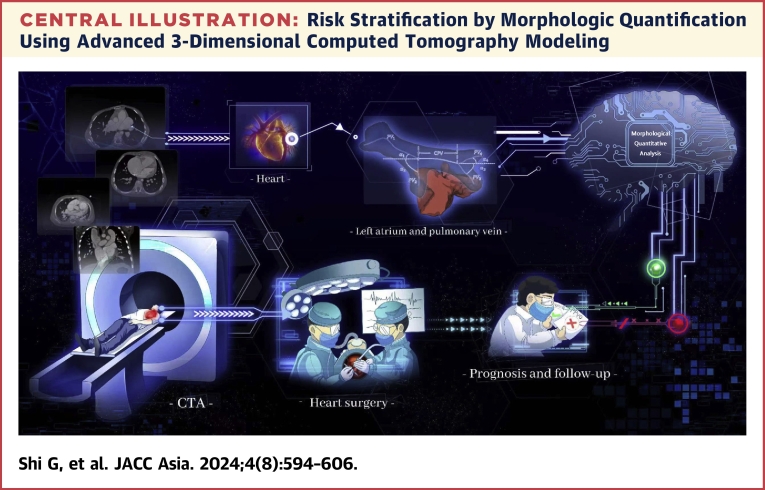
Risk Stratification by Morphologic Quantification Using Advanced 3-Dimensional Computed Tomography Modeling Morphologic quantification derived from advanced 3-dimensional computed tomography modeling assists in individualized risk stratification. CTA = computed tomography angiography.
